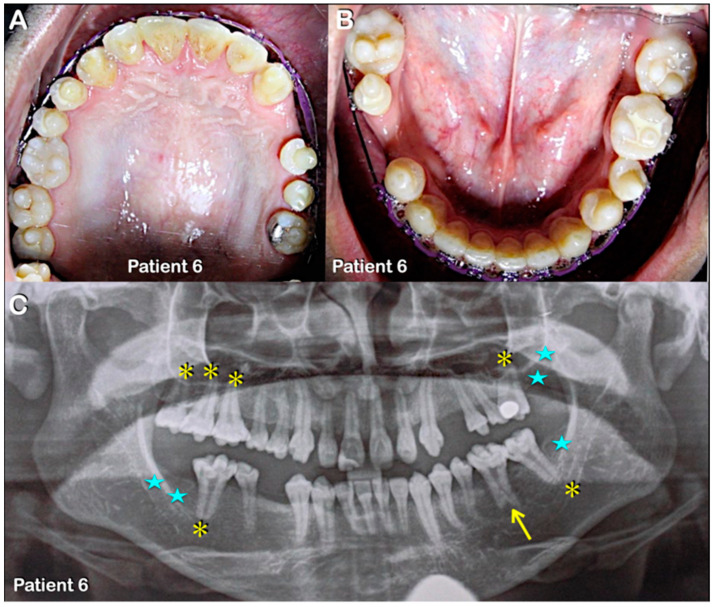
Patient 6. (A–C) Patient 6. (A,B) Permanent dentition. Round-shaped permanent molars, molars with multiple supernumerary cusps, and premolars with single prominent cusps. (C) Panoramic radiograph showing agenesis of second and third permanent molars (blue stars) and single-rooted permanent molars (yellow asterisks). Note severe taurodontism (yellow arrow).
